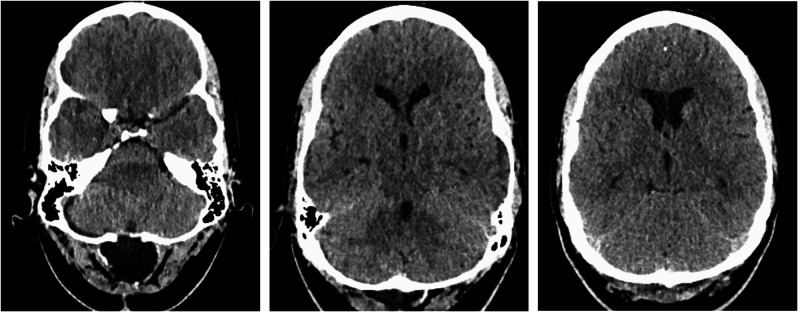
CT head after posterior fossa re-exploration surgery. Repeat CT head demonstrating almost complete resolution of the posterior fossa subdural hygromas after surgical drainage and mild hydrocephalus.
